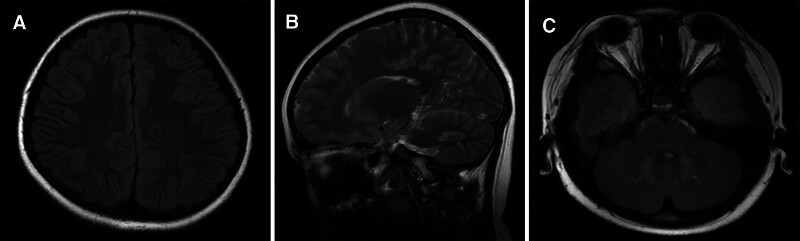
Cranial MRI showed that there were abnormal signs (White arrow) in right frontal. (A) and left parietal areas as well as symmetrical abnormal signals in bilateral cerebellum. (B and C) and corpora quadrigemina. MRI = magnetic resonance imaging.
